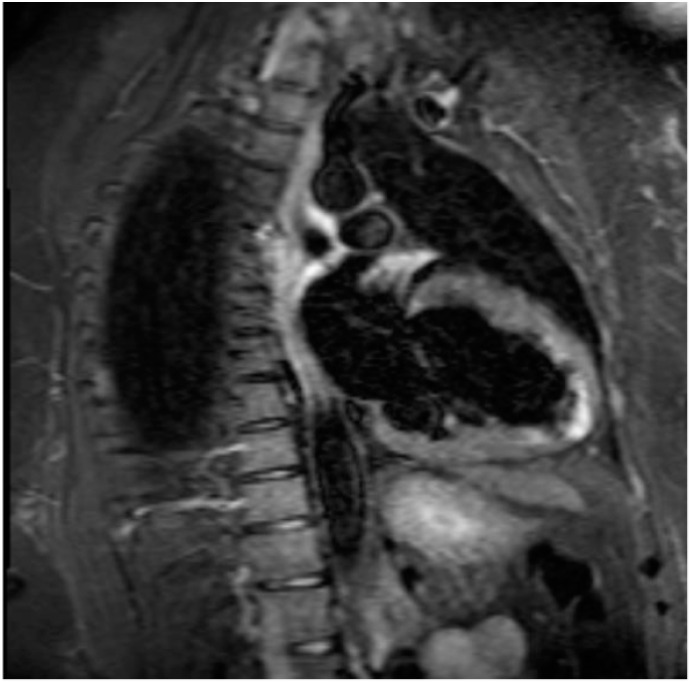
Regional myocardial edema in COVID-19—T2-weighted imaging.
